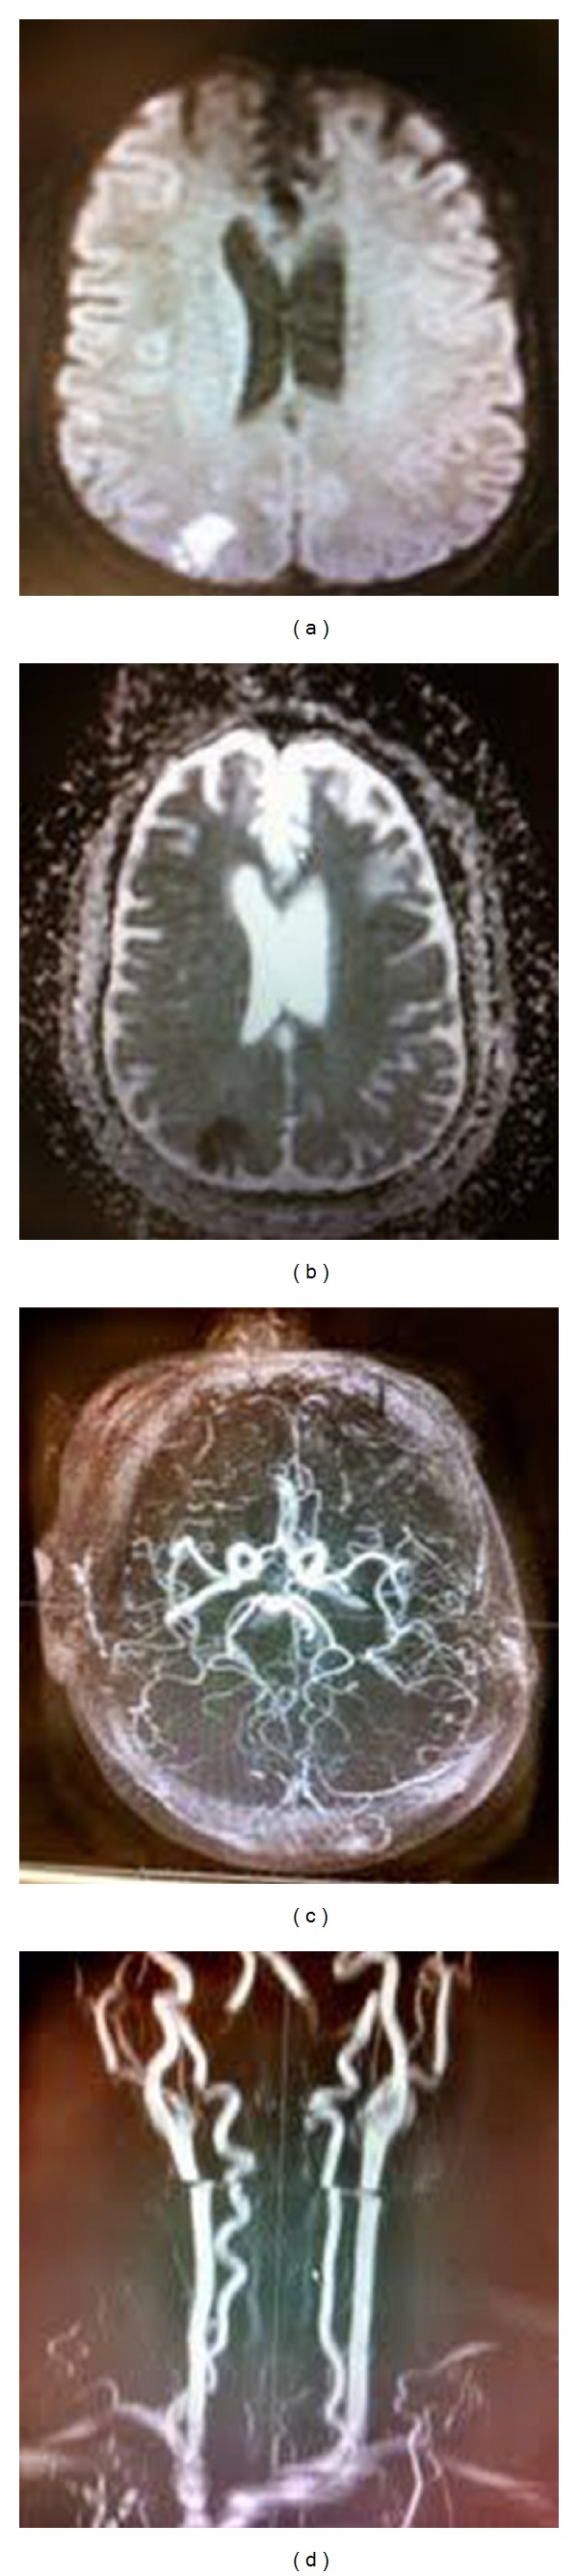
MRI Brain (a) DWI showing hyperintense lesion in the right MCA territory. (b) ADC showing mismatch in the same region confirming acute infarction. (c) and (d) MRA showing no evidence of thrombus formation or carotid dissection.
